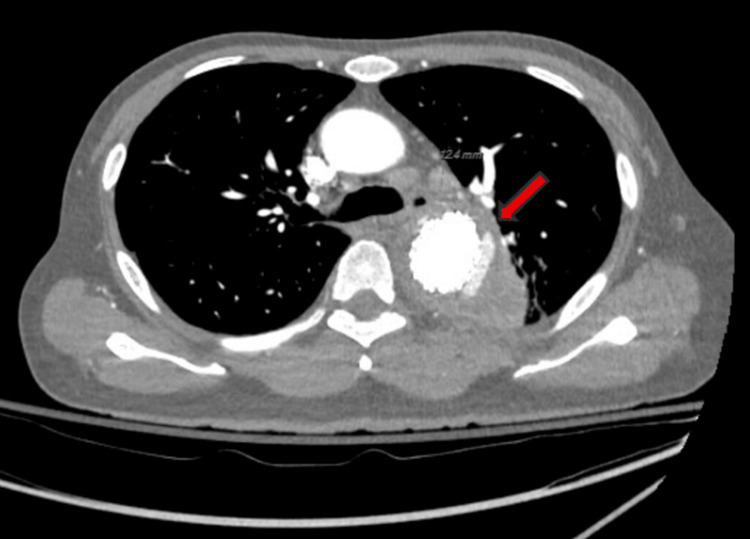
CTA chest at 24 weeks demonstrated a new contained aortic perforation with associated intramural hematoma consistent with pseudoaneurysm formation, likely secondary to tuberculous aortitis (TA) Axial contrast-enhanced CT demonstrating an endovascular stent graft within the descending thoracic aorta with a new dissection and contained perforation at the proximal landing zone measuring approximately 0.8×3.1×5.3 cm. Crescentic hyperdense blood products extending beyond the dissection flap are consistent with intramural hematoma. Associated aneurysmal dilation of the descending thoracic aorta is also noted.
